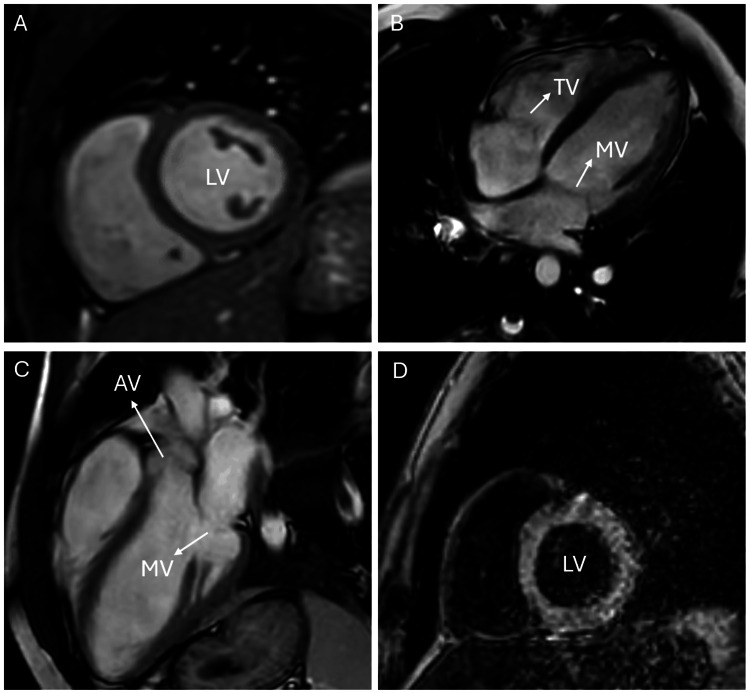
MRI Cardiac morphology function Multiple planes in cardiac MRI showing normal appearances. (A) The short-axis view shows no (LV) left ventricular wall abnormalities (B) The four-chamber view shows normal (MV) mitral and (TV) tricuspid valves (C) The left ventricular outflow tract view shows normal (AV) aortic and (MV) mitral valves. (D) The Post contrast short-axis view shows normal enhancement pattern.
